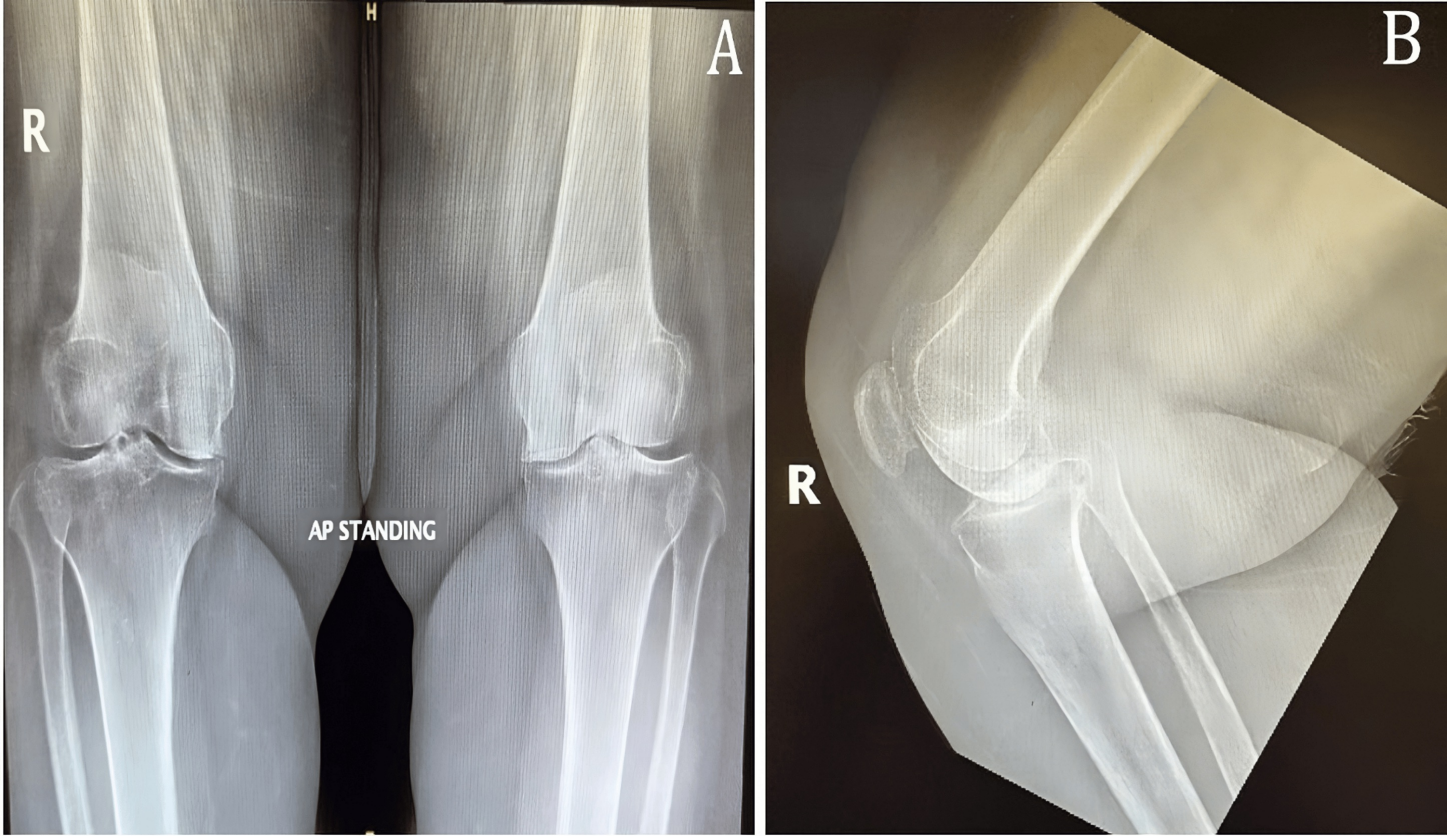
(A) Bilateral standing knee AP view X-ray preoperatively. (B) Right knee lateral view X-ray preoperatively AP: anteroposterior
